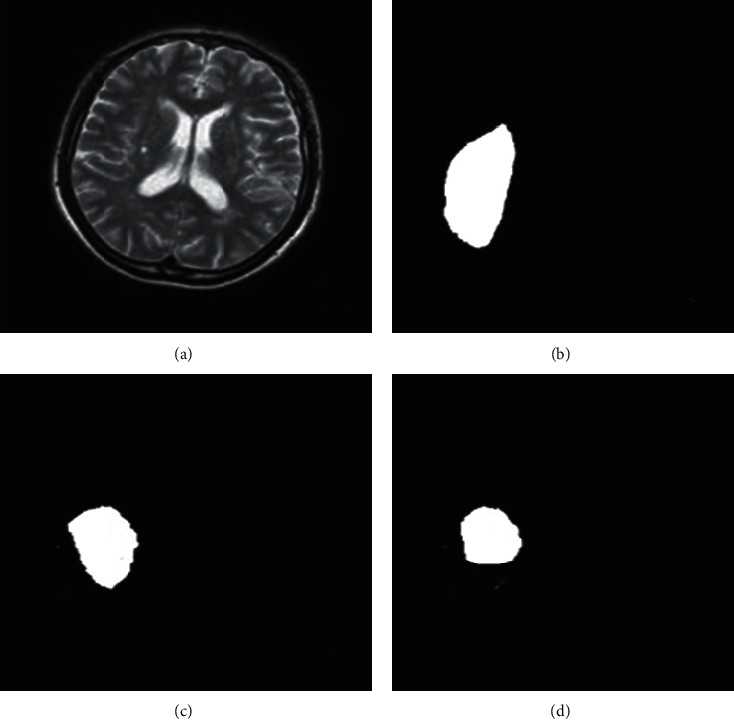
Comparison of the segmentation effects of the three algorithms. (a) Magnetic resonance images of patients with severe stroke were input; (b) FCN algorithm segmentation effect; (c) segmentation effect of U-Net algorithm; (d) segmentation effect of the proposed algorithm.
